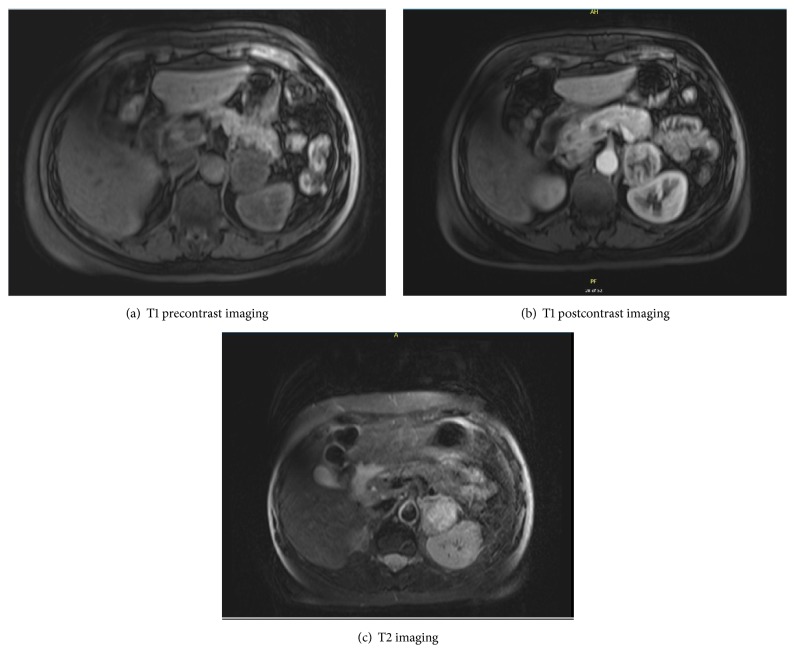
MRI abdomen with and without contrast. A 3.4 x 4.0 x 4.7 cm mass is seen in the left adrenal gland. The lesion demonstrated heterogeneous T2 hyperintense/T1 isointense to muscle signal. The T1 signal of the lesion was hypointense relative to the contralateral right adrenal gland.
